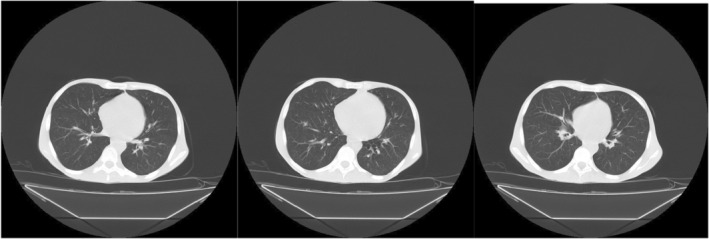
Chest CT scan. There was no abnormal finding.
